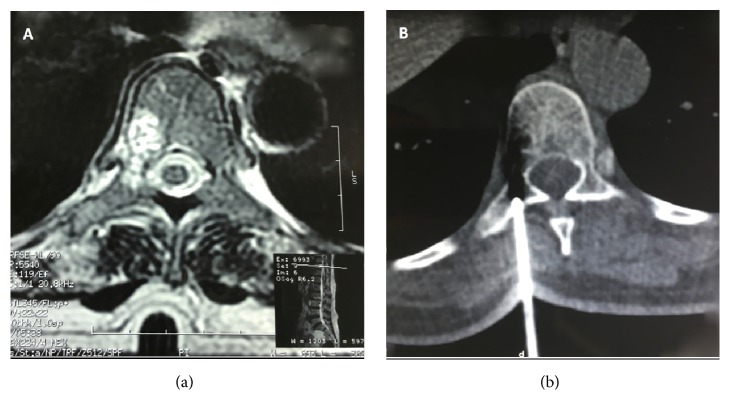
(a) MRI showing an abnormal signal blowing the cortical of the D10 vertebral body. (b) scan guided biopsy of the suspected lesion.
